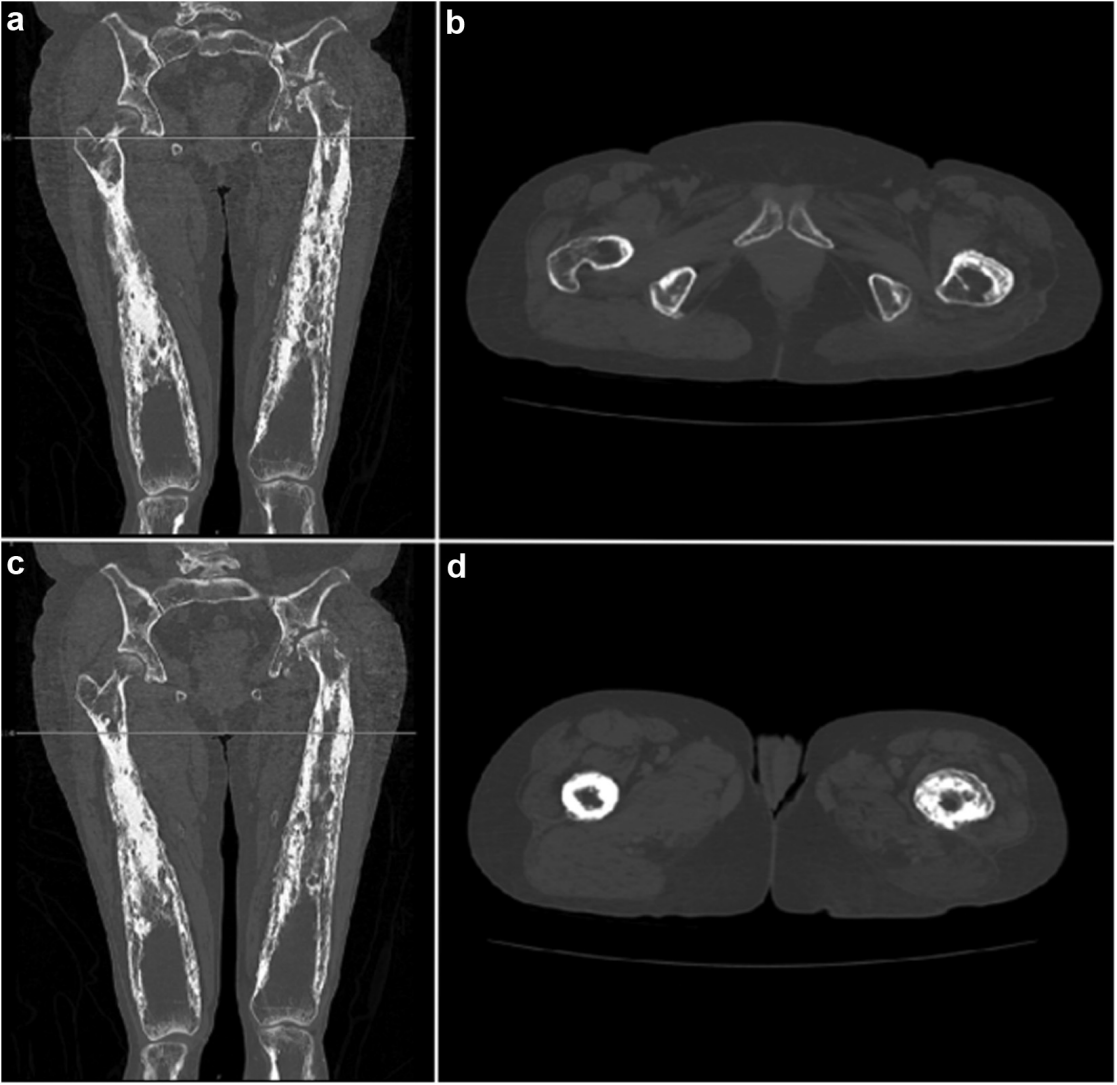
Preoperative computerized tomography (CT) scans in the coronal (a, c) and axial (b, d) planes demonstrating diffuse cortical thickening and narrowing of the medullary canal.
